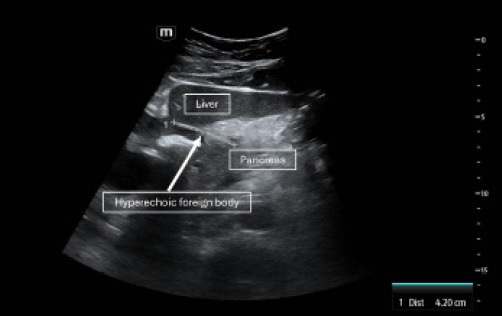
Gray-scale transverse routine point-of-care ultrasound image of the pancreas showing the foreign body (dotted line/arrow) in a patient diagnosed with foreign body-induced pancreatitis.
